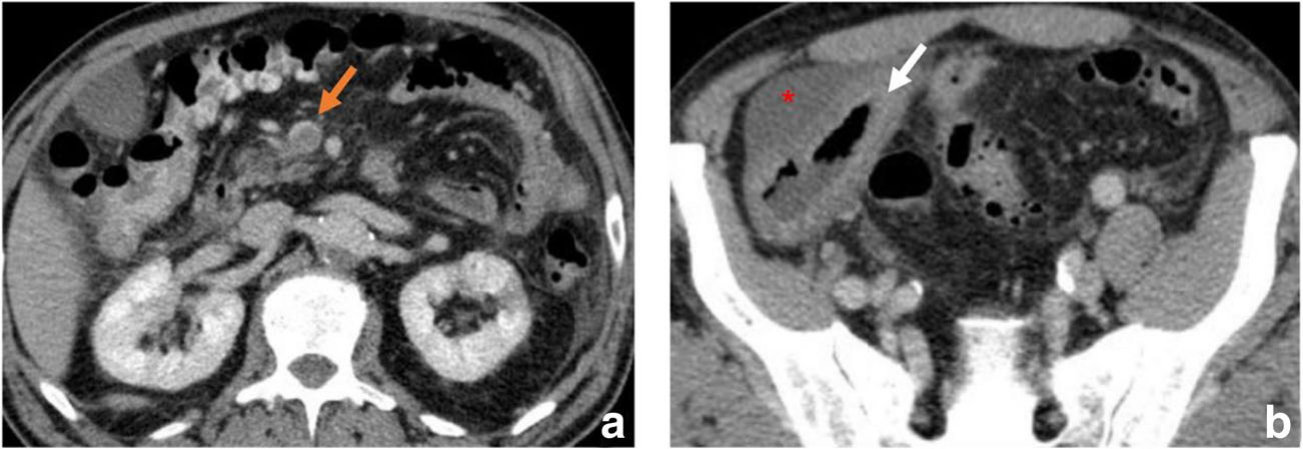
Venous occlusion. Contrast-enhanced CT image of the abdomen in 49-year-old female with superior mesenteric vein thrombosis. (a) Contrast-enhanced axial CT scan shows a thrombus in the superior mesenteric vein in axial plane (orange arrow); (b) wall thickening of the ascending colon (white arrow). Ascites (*) is also visible
